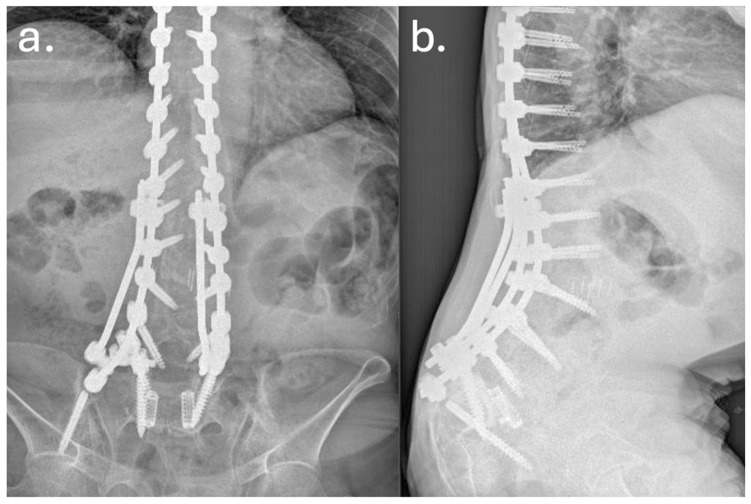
Anteroposterior (a) and lateral (b) radiographs at 2-year follow-up. The images demonstrate loosening of the bilateral iliac screws with peri-implant radiolucency, consistent with mechanical failure at the lumbopelvic junction. The sagittal view shows partial loss of the achieved lumbar lordosis and persistent sagittal imbalance, suggesting progressive biomechanical compromise of the construct despite maintenance of overall instrumentation continuity.
